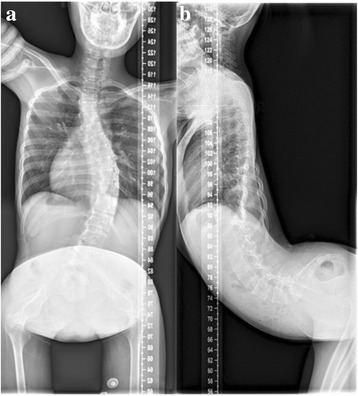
Preoperative anterior-posterior and lateral X-ray of whole spine. a Anterior-posterior X-ray showed Cobb of scoliosis (T5-L2) is 44°. b Lateral X-ray showed Cobb of thoracolumbar lordosis (T9-S1) is 116°
